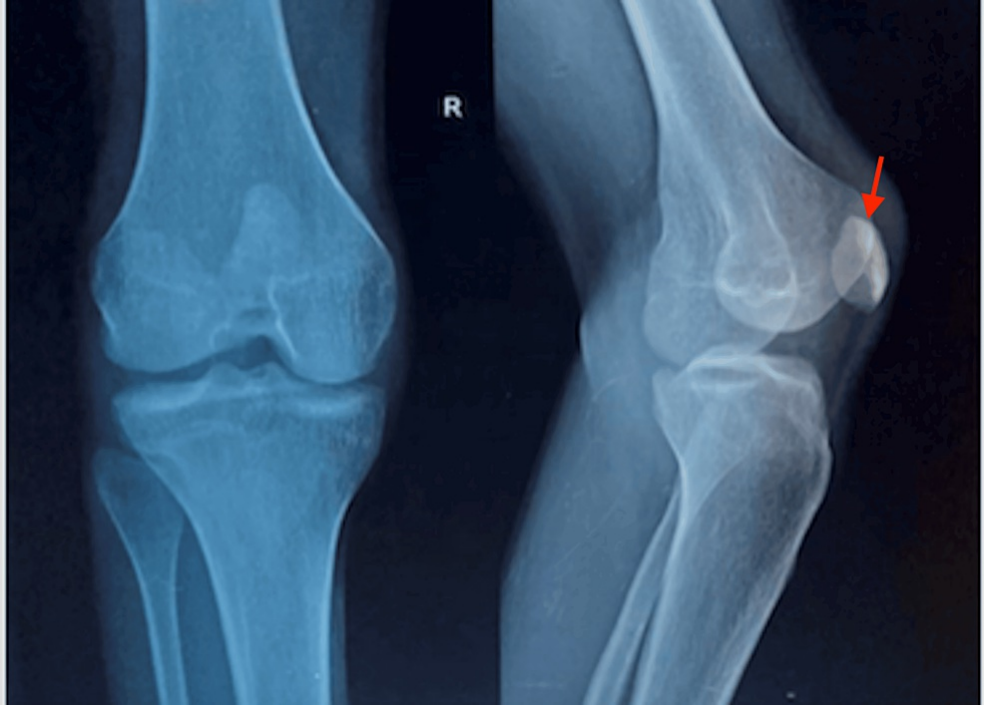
Bilateral knee X-ray showing hypoplastic and dysplastic patella
